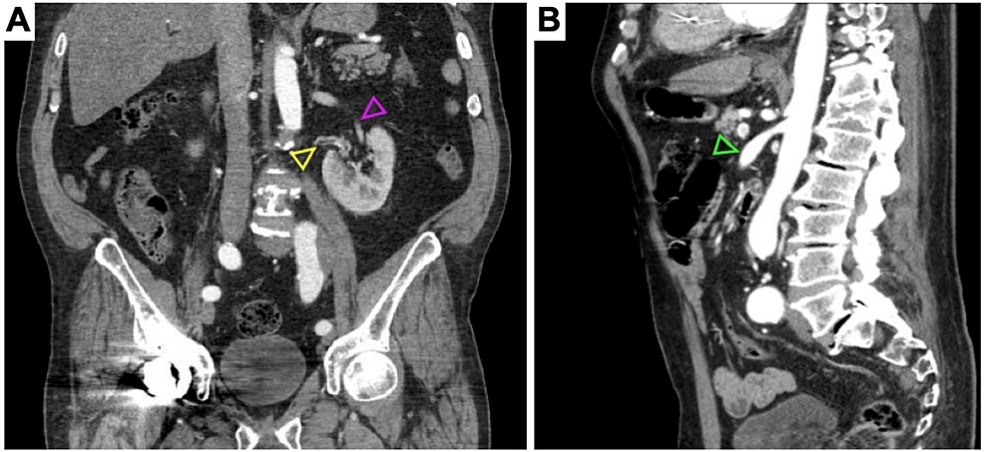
Accessory renal arteries and superior mesenteric artery aneurysm. CT angiograms of the abdomen and pelvis showing (A) the dominant left renal artery (purple arrowhead) arising at the level of the L2 vertebrae, an accessory left renal artery (yellow arrowhead) arising more inferiorly from the descending aorta at the level of L3-L4, and (B) a 1.4-cm aneurysm of the proximal superior mesenteric artery (green arrowhead).
